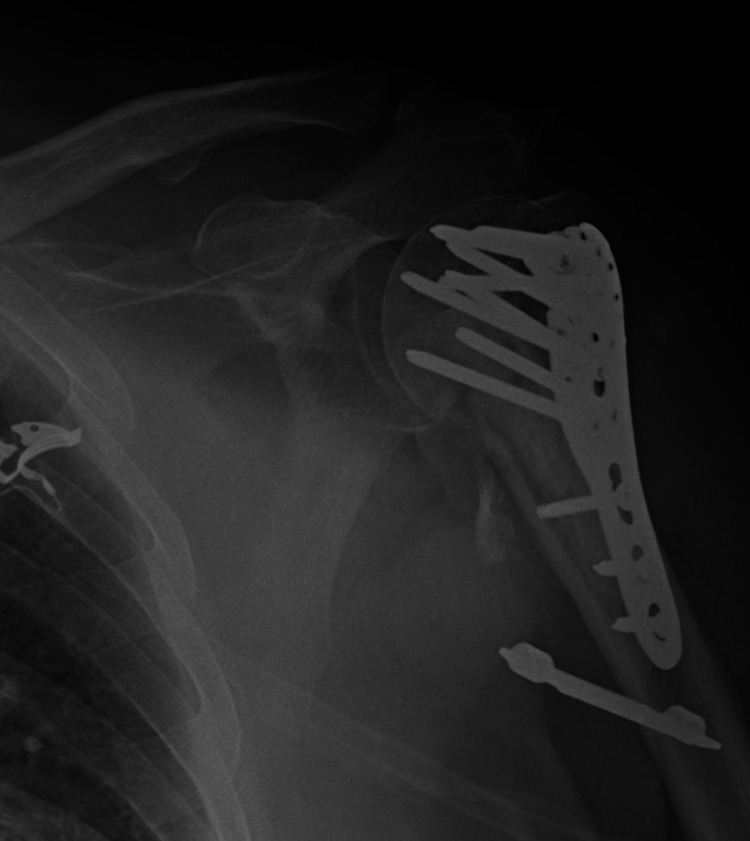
Postoperative anteroposterior (AP) radiograph of the left shoulder status post open reduction and internal fixation (ORIF) with fibular strut augmentation.
